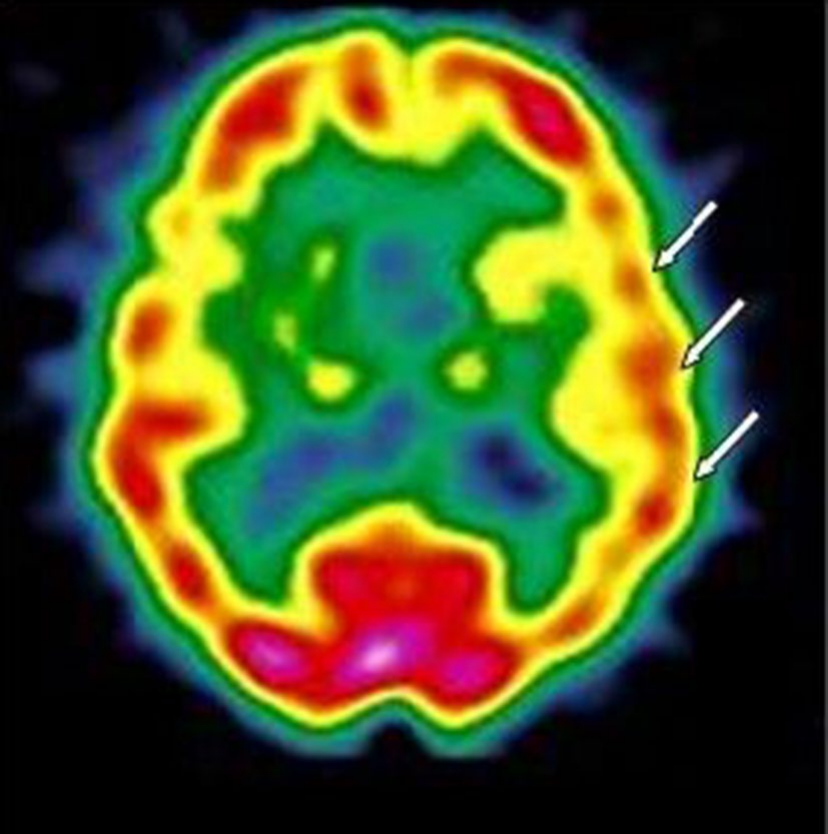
Case # 2. ^99m^Tc-ECD brain SPECT: hypoperfusion in the left temporal and parietal region (*arrows*)
